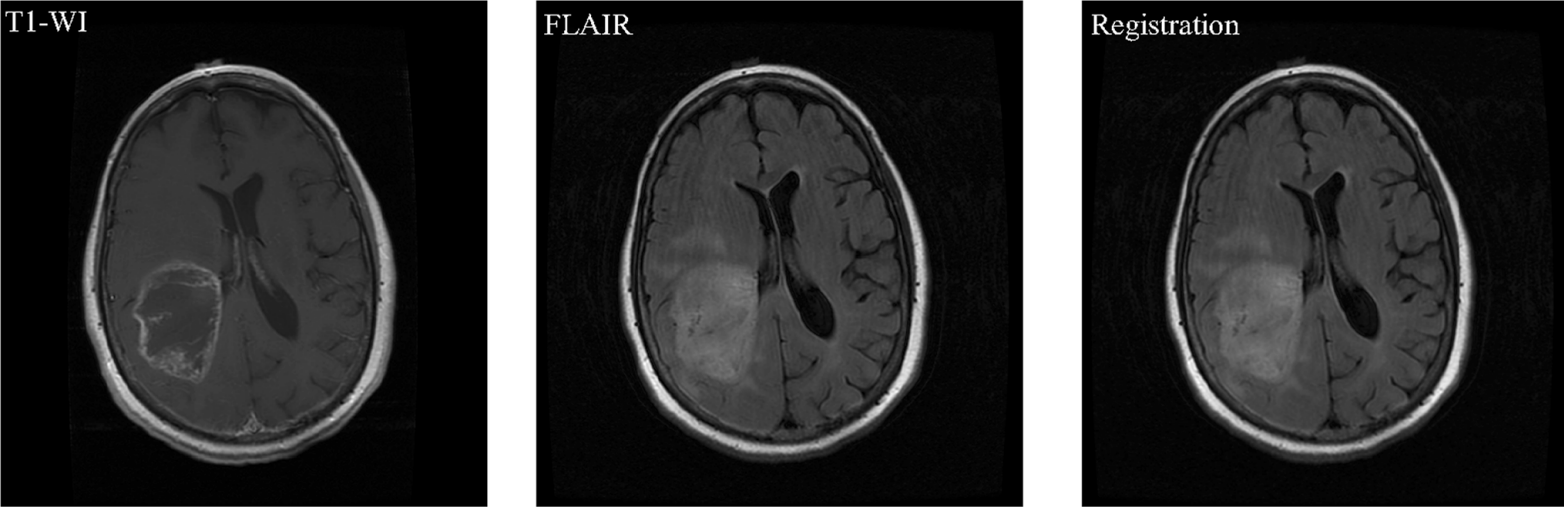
Example of axial brain image with resembles intensity value of both skull and tumor. Brain image within tumor on T1-WI, the corresponding axial FLAIR sequence, and the corresponding rigid registration based on fixed image T1-WI and moving image FLAIR sequence
